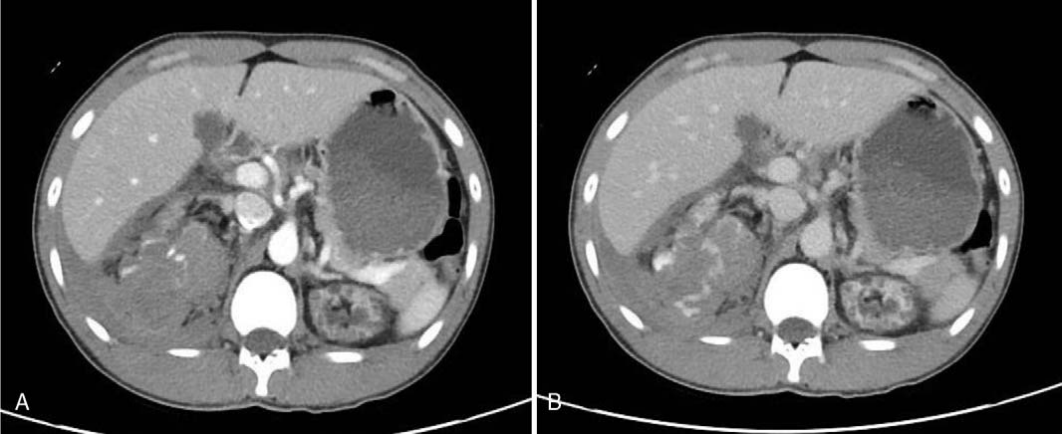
Contrast-enhanced CT showing a right perirenal hematoma with pseudoaneurysm, retrohemoperitoneum, and hemoperitoneum. (A) Portal phase. (B) More contrast dye leakage was observed on delayed-phase imaging.
